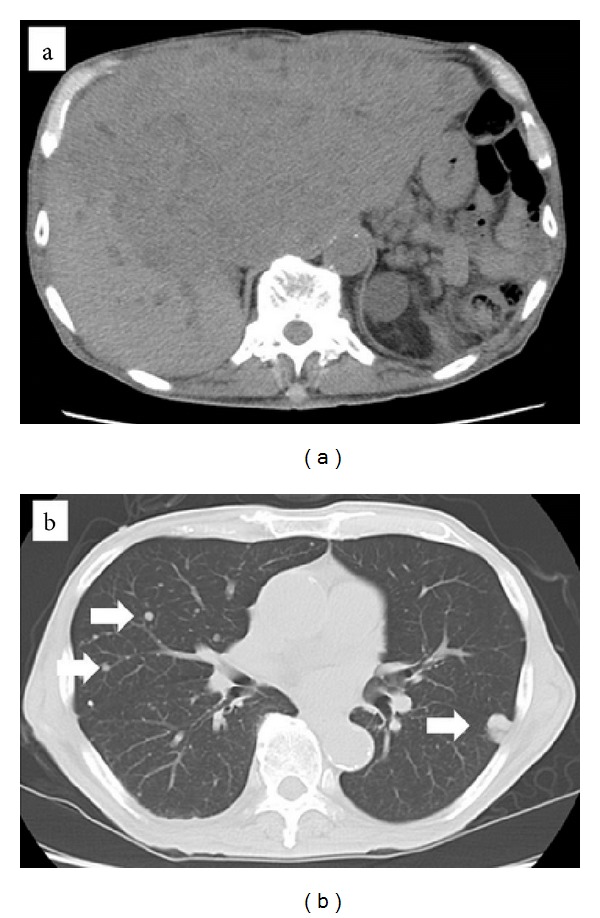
Computed tomography (CT) of the abdomen and chest. Multiple liver metastases (a) and lung metastases ((b) arrows) were detected on CT.
